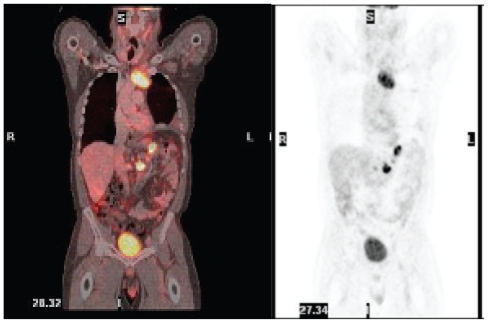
PET-CT demonstrates uptake in mediastinal mass, and two foci close to proximal small intestine.
